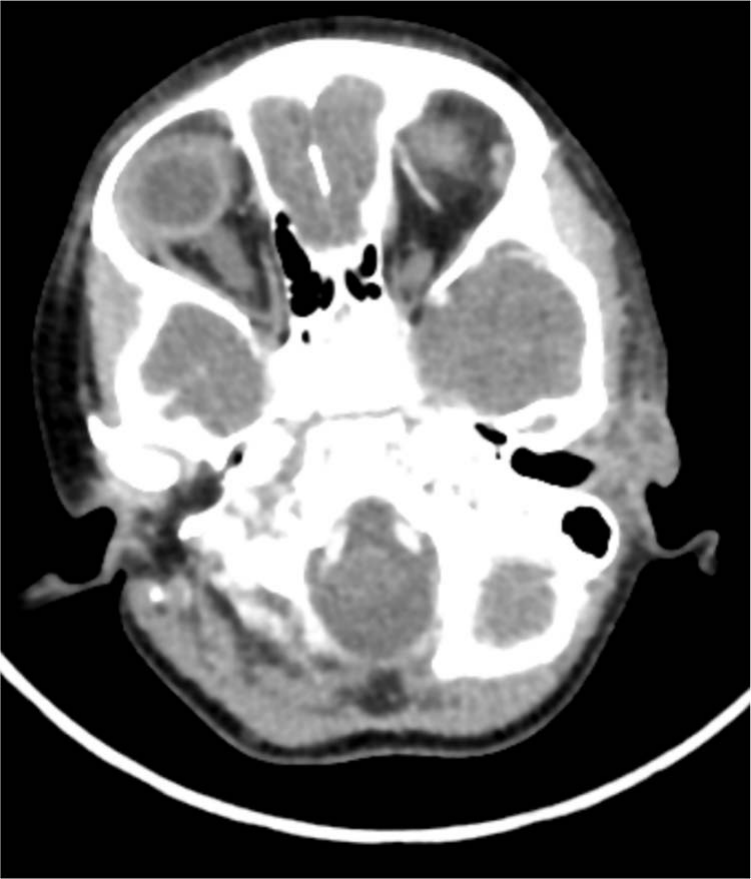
This is the axial cut CT that showed left-sided cystic lesion representing infected preauricular sinus. The CT did not show the second branchial cyst or tract that was found in clinical examination.
